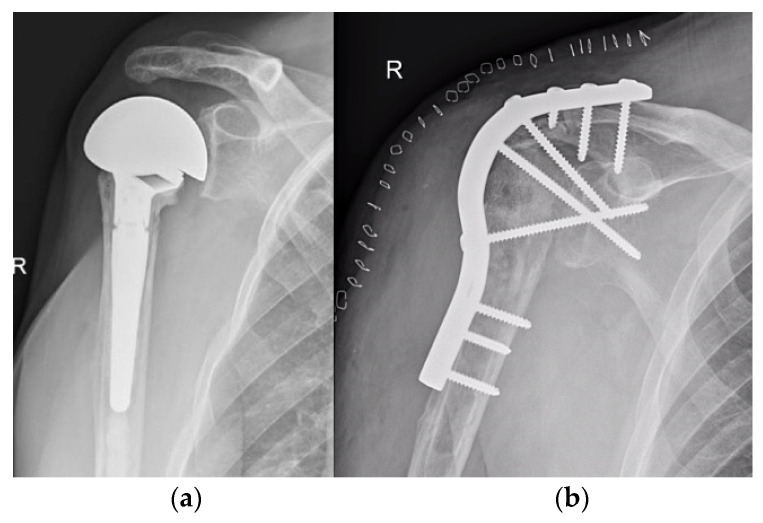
Shoulder arthrodesis. (a) preoperative X-ray after failed shoulder arthroplasty; (b) postoperative X-ray after shoulder arthrodesis.
